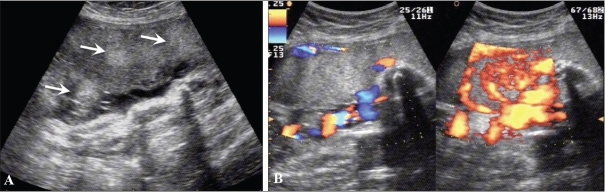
25-weeks' gestation with a rather bulky placenta. Note the focal hyperechoic areas (arrows in A) in an anteriorly located placenta. Color Doppler is not of great help but power Doppler (B) demonstrates the abnormal vascularity in this chorioangioma (image on the right)
